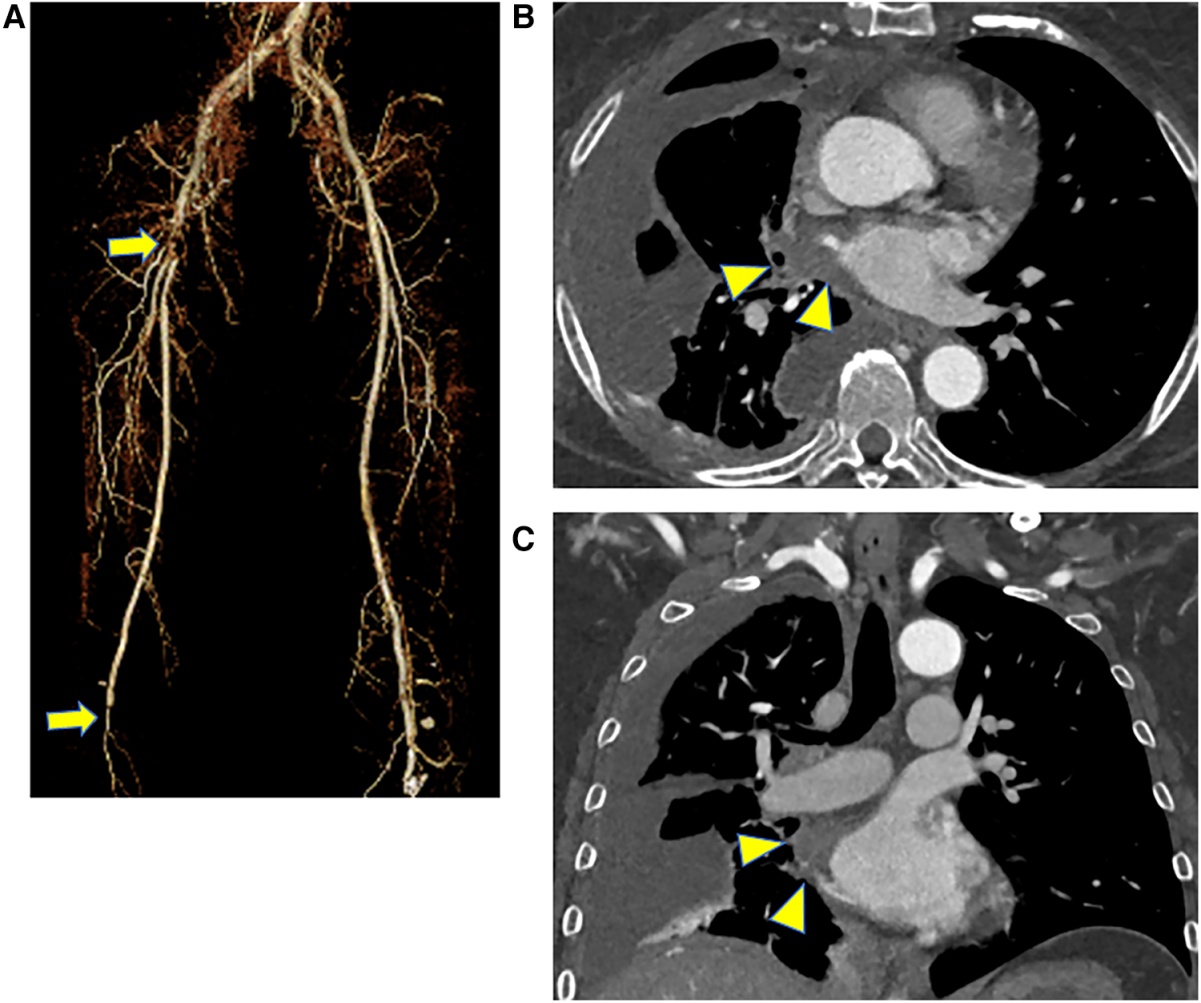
Preoperative CT scans, (A) three-dimensional CT of the lower extremity, and (B,C) CT of the chest level. CT showed contrast deficits from the right CFA to the proximal SFA and DFA and at the popliteal artery behind the knee (arrow). There was also a contrast defect from the right pulmonary vein into the left atrium, which might be a thrombus with a direct invasion of pulmonary metastases (arrowhead). CT, computed tomography; CFA, common femoral artery; SFA, superior femoral artery; DFA, deep femoral artery.
